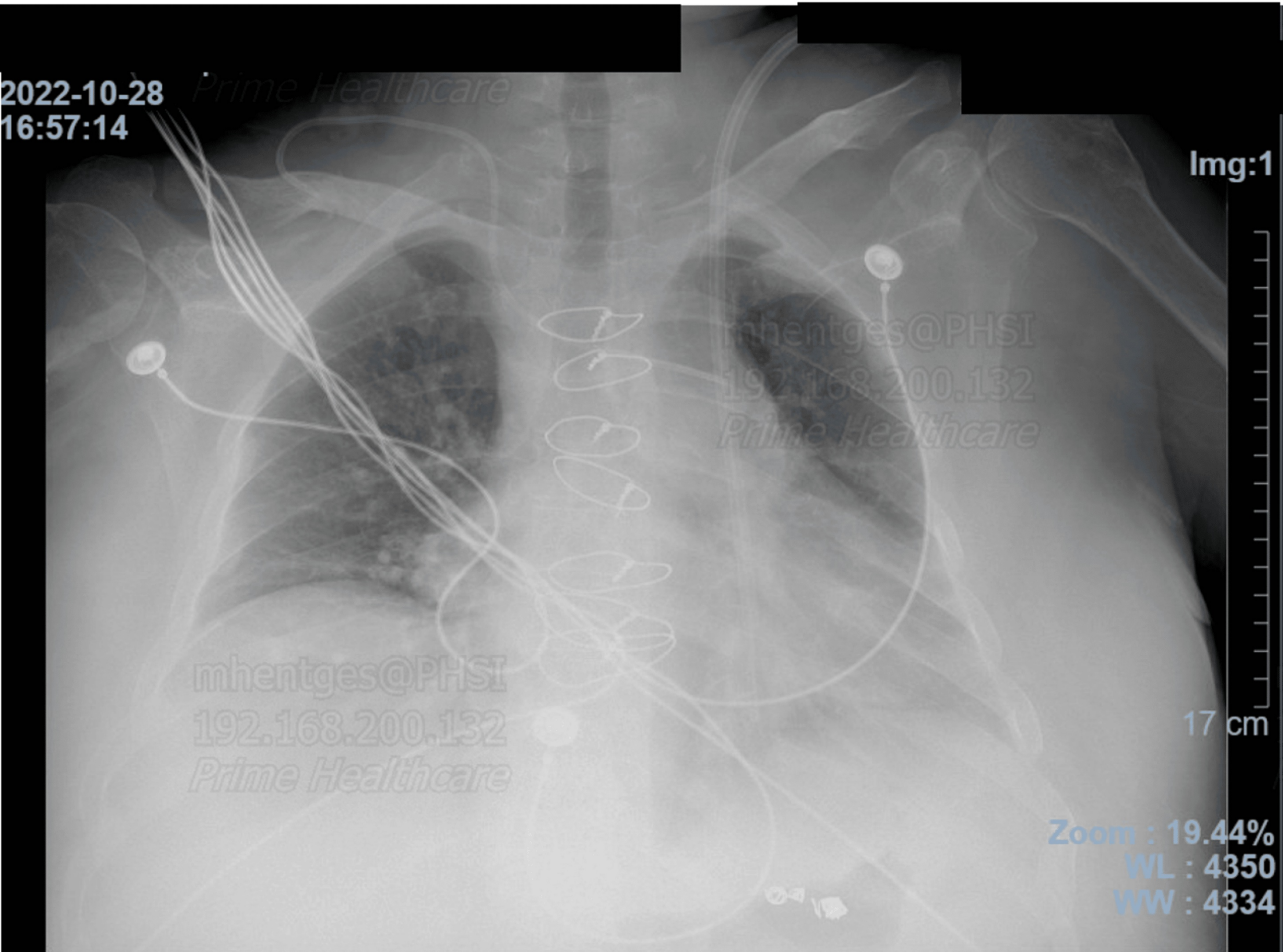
Chest X-ray, 2022. The temporary dialysis catheter was visualized descending down the left chest via a left-sided SVC dumping into the coronary sinus. SVC: superior vena cava
